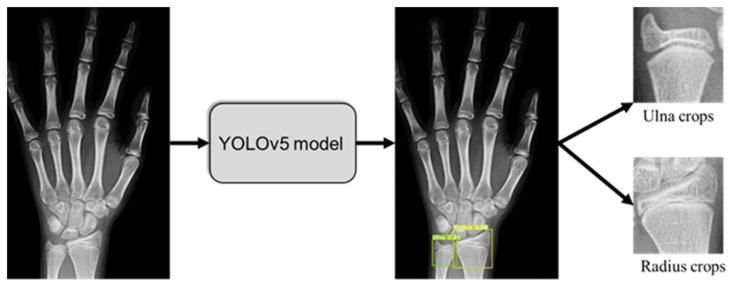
DRU object detection from hand X-rays and crops extraction.
